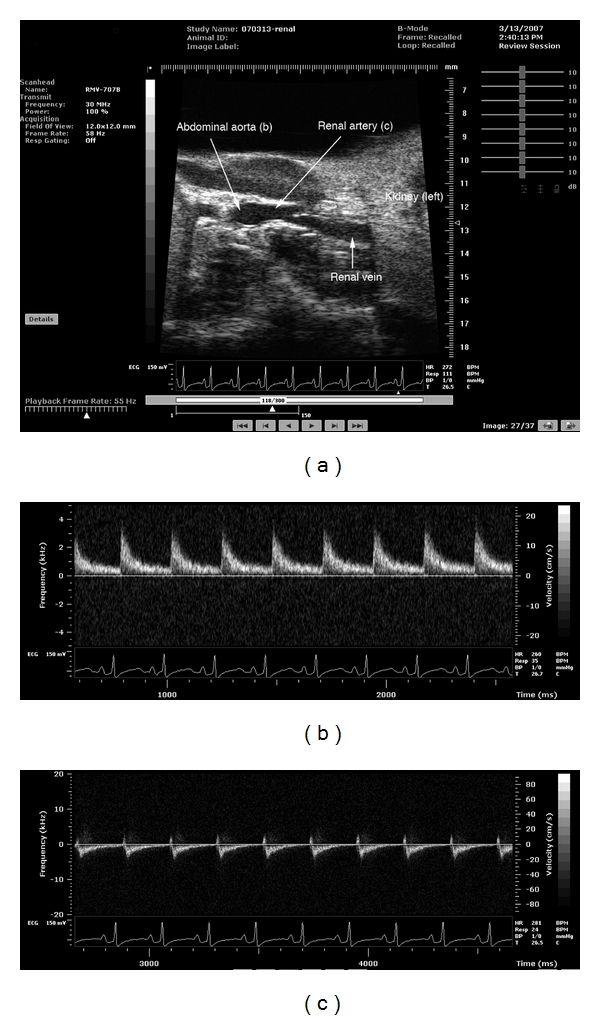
Ultrasound microimaging around the left kidney (a) and Doppler flow measurement at the abdominal aorta (b) and renal artery (c). Appropriate positions of the abdominal aorta, renal artery, and renal vein observed with the ultrasound imaging device are indicated in (a). The blood flow of the abdominal aorta indicated by the arrow in (a) is shown in (b). The blood flow of the renal artery indicated by the arrow in (a) is shown in (c). Doppler flow data show unidirectional (b) and oscillating (c) flow patterns in the abdominal aorta and renal artery, respectively. Note that the waveform of the renal artery indicated in (c) is the opposite of that for the abdominal aorta in (b) because of the position of the ultrasound probe.
